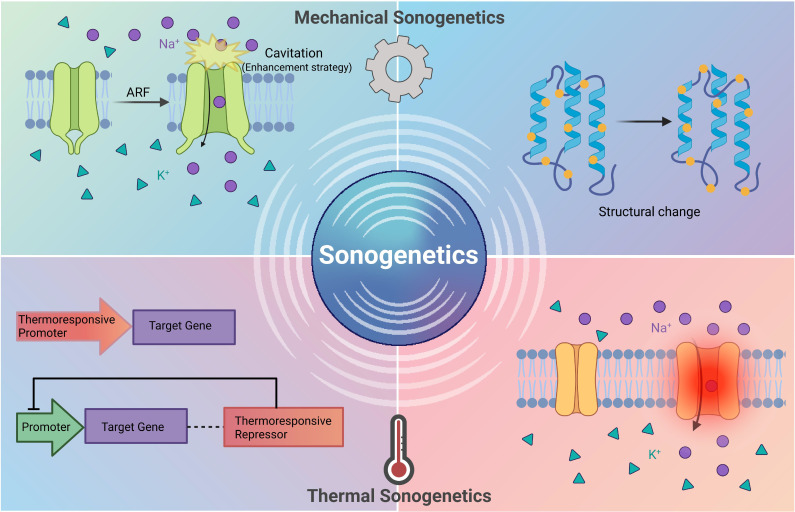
Classification of sonogenetics. Mechanical sonogenetics utilizes ARF and cavitation. Ultrasound directly triggers conformational changes of the exogenously expressed MS ion channels in target cells, producing a series of biological effects. Thermal sonogenetics uses thermal energy generated by ultrasound to activate thermoresponsive elements, such as heat-shock promoters or thermoresponsive repressors, to regulate gene expression. Created with BioRender.com. ARF: acoustic radiation force; MS: mechanosensitive.
